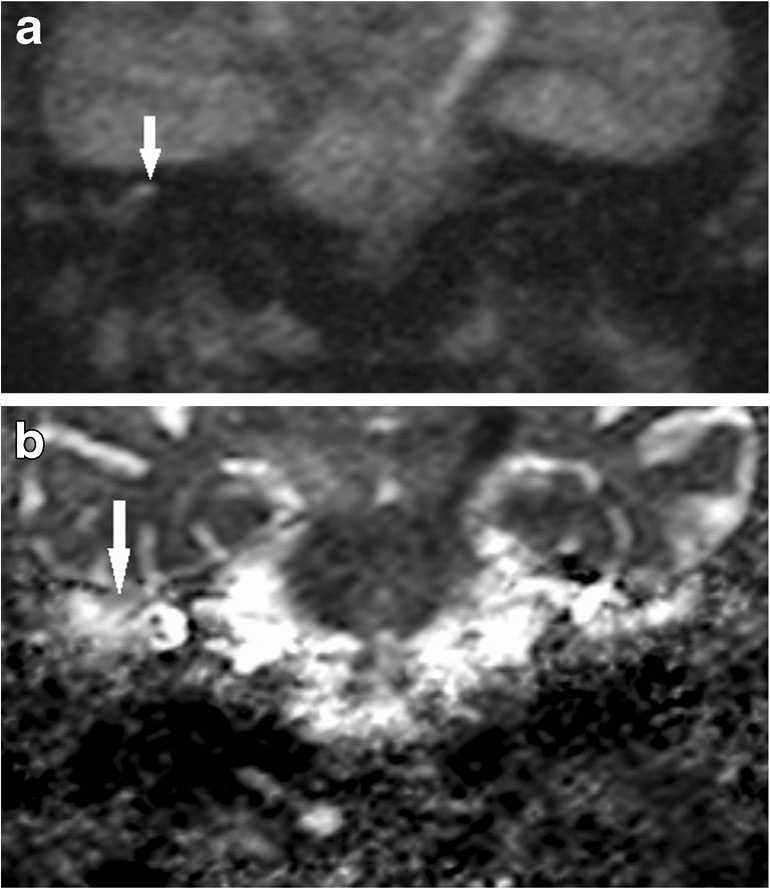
False positive high DWI signal for cholesteatoma. Non-cholesteatomatous tissue (surgically confirmed non-specific inflammation) at the post-operative mastoid remnant, which demonstrated a high signal (*white arrow*) on the b1000 image suggestive of residual cholesteatoma. However, b the corresponding ADC map demonstrated borderline high ADC signal (*white arrow*) and value *not supporting* the diagnosis of residual cholesteatoma. This case could also have been resolved with a follow-up DWI scan
